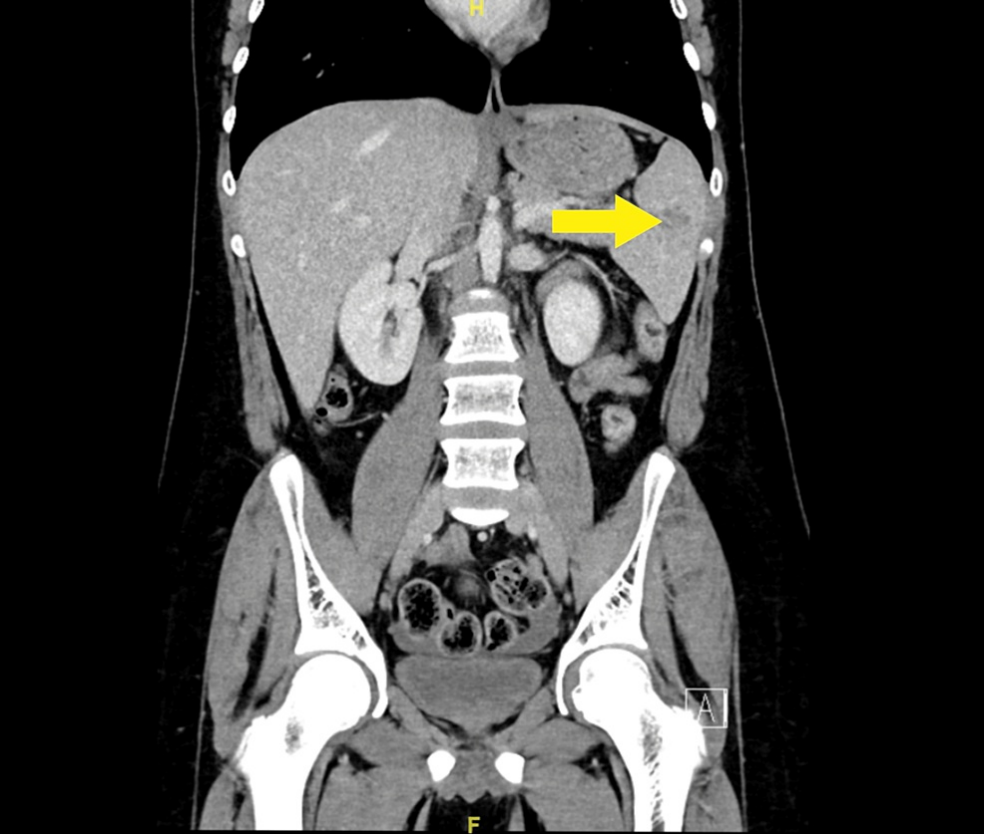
Coronal view of contrast-enhanced computed tomography of abdomen suggesting grade 2 splenic lacerations showing multiple linear hypo-density extending from the splenic hilum reaching up to 2.5 cm.
